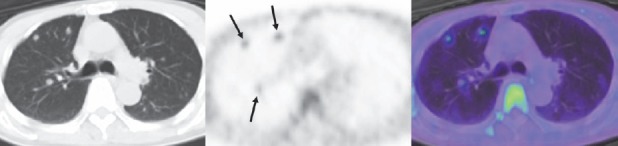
Axial images of the chest in the patient presented in Figure 1. From left to right, a CT image of anti-^18^F-FACBC PET/CT, PET image, and PET/CT fusion image are presented. Increased uptake is shown in tiny lung nodules (arrows). The same scale was used for presentation of PET in this figure as in Figure 1
